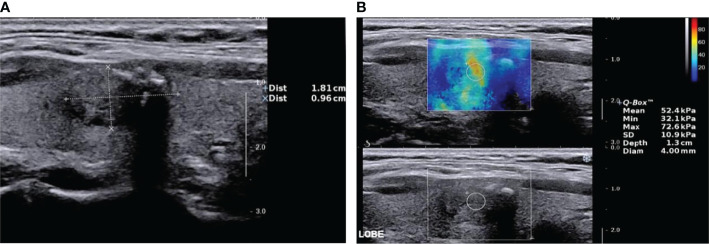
Nodular goiter (shear wave elastography false-positive nodule) by two-dimensional ultrasound and elastic ultrasound. (A) A solid isoechoic nodule in the middle of the right lobe of the thyroid, 18.1 × 9.6 mm, with an ill-defined margin and coarse calcification. The ACR–TIRADS score was 4 and ACR–TIRADS category was 4. (B) The Emax value of the nodule on SWE imaging was 72.6 kPa.
